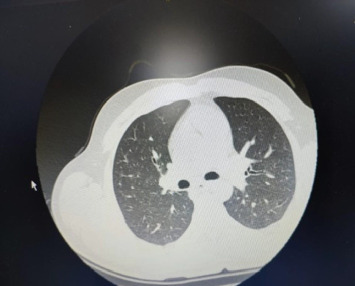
Chest CT scan revealing no signs of infection or notable abnormalities, suggesting the absence of pulmonary involvement.
